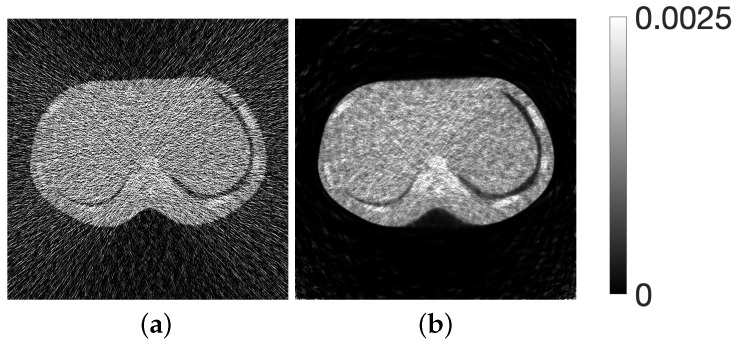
Images reconstructed from X-ray CT scanner projections using (a) FBP procedure and (b) MLEM method.
